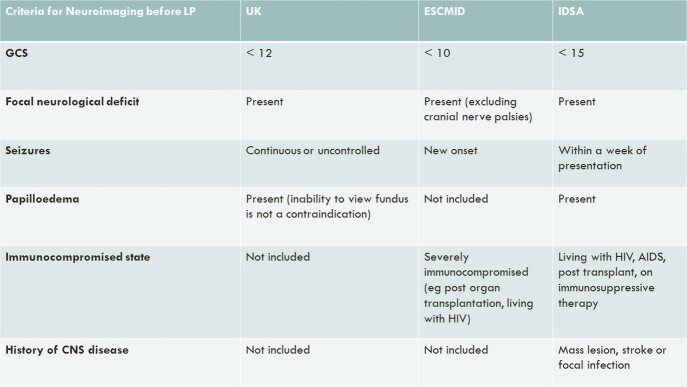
Criteria for CT Brain before Lumbar Puncture
